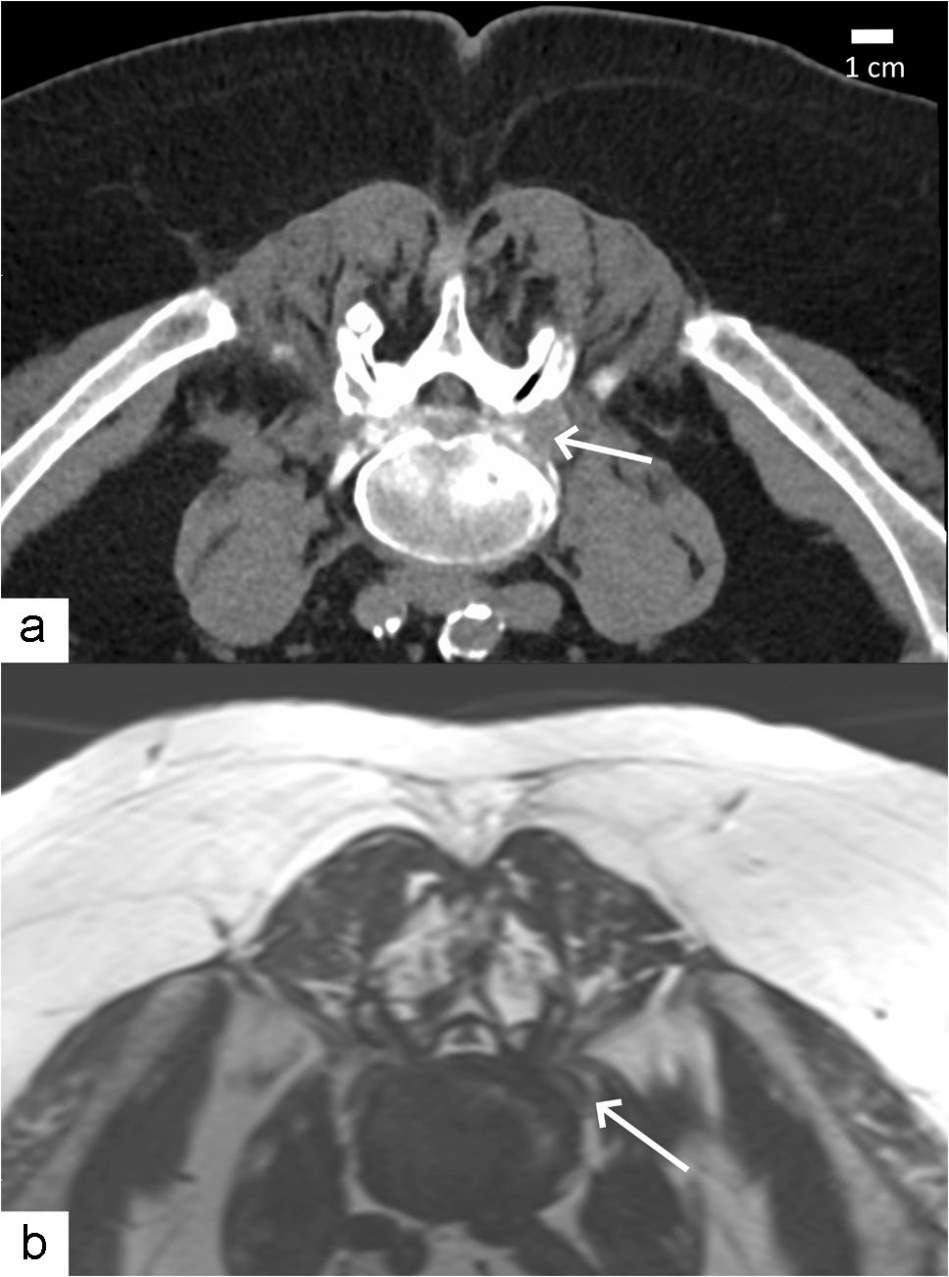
Non-contrast CT (a) and MRI (b) scans obtained in a 64-year-old man with left L5 radiculopathy, secondary to L5 antelisthesis, and severe L5-S1 neural foraminal narrowing. a Axial oblique CT image showing fibrotic changes inside the neural foramen (arrow), thwarting a clear delineation of exiting nerve. b Axial oblique proton density-weighted MRI image clearly depicting the nerve (arrow)
